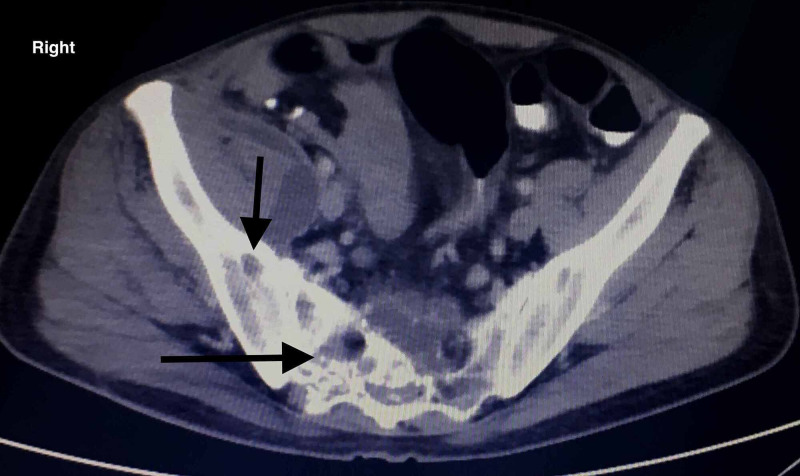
CT evaluation of the pelvis. The sacrum and the right sacroiliac joint are both affected with noticeable corrosions.
